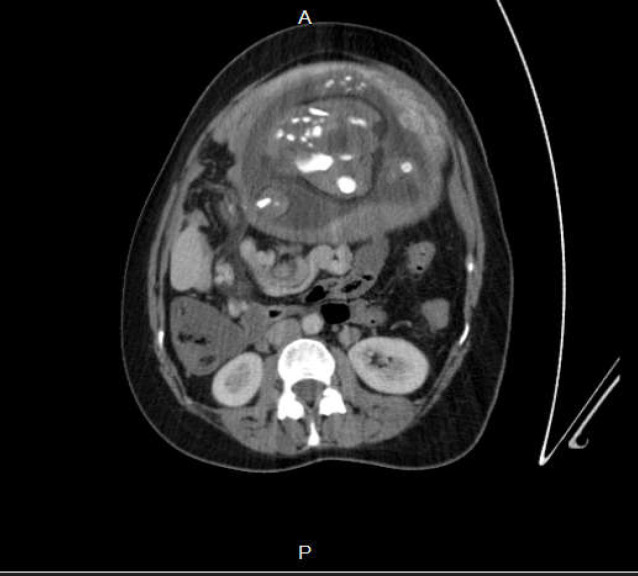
Absence of short bowel and mesothelium in right side of CT represents a term known as mesothelium stalk
